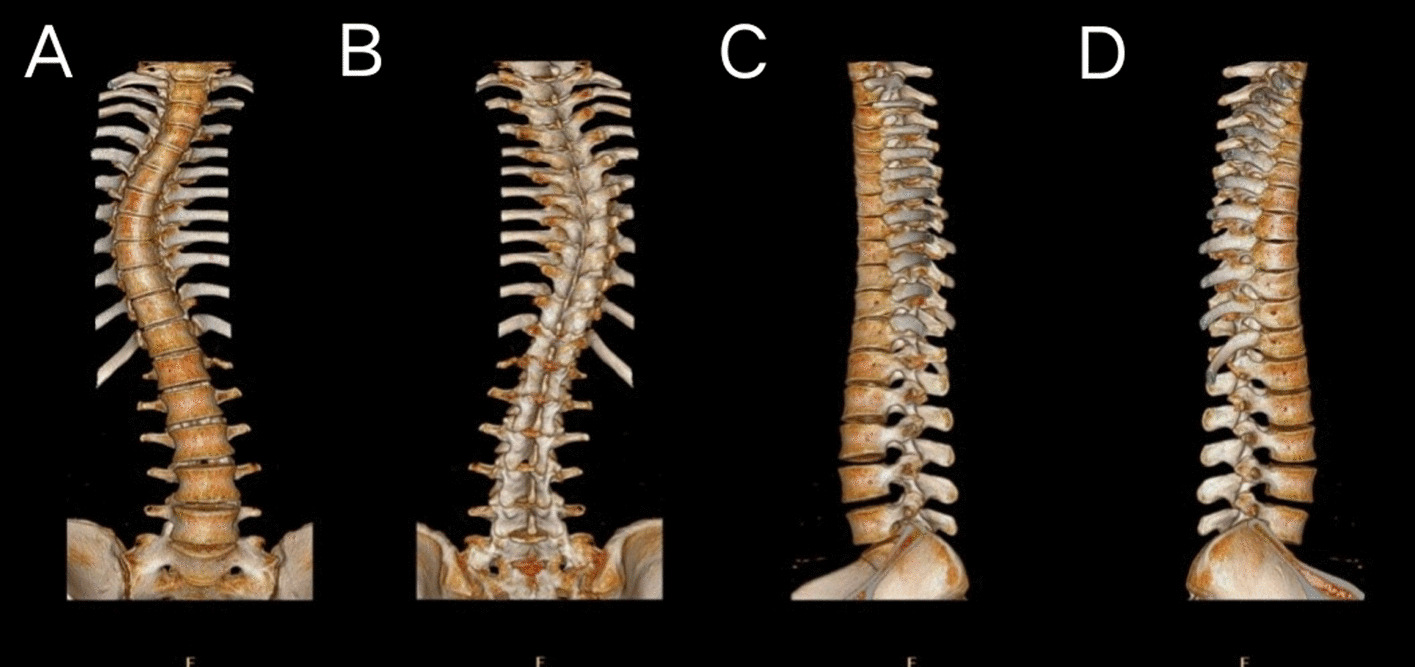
3D imaging of an 18-year-old patient with idiopathic scoliosis generated by processing CT images. A Anterior view B Posterior view C Left lateral view D Right lateral view
